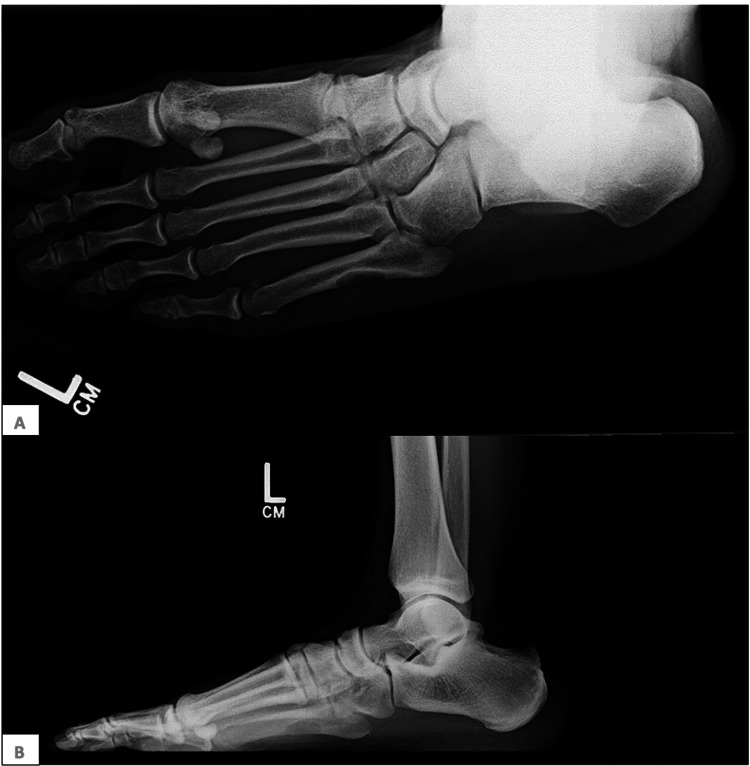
Pseudomyogenic hemangioendothelioma X-rays of ovoid, cortically based lesion at the proximal fifth metatarsal shaft. (A) Anterior view; (B) lateral view.
